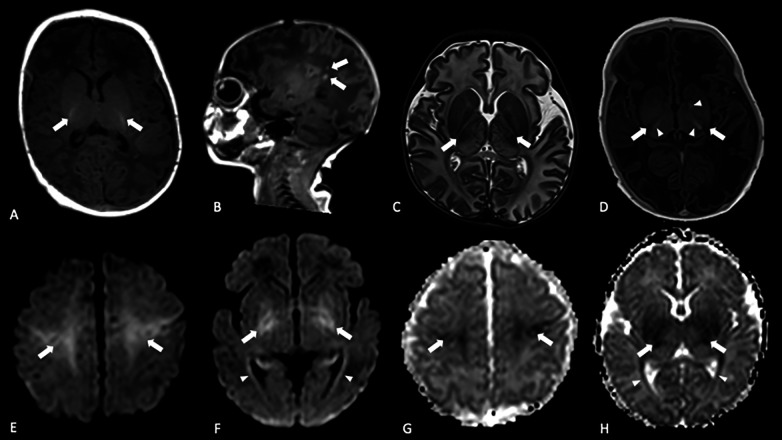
Brain MRI in term HIE neonates treated with TH: (A) axial 3D GrE T1w showing the physiological PLIC hyperintensity (arrows); (B) sagittal 3D GrE T1w demonstrating focal areas of high signal due to PWMLs (arrow);(C) axial TSE T2w showing the absence of the typical PLIC hypointensity (arrows); also, note the abnormal thalamic signal; (D) axial IR T1w showing the loss of the typical PLIC hyperintensity (arrows) and exaggerated hypersignal in the thalami and left globus pallidus (arrowheads); (E,F) axial DWI and (G,H) the corresponding ADC maps showing restricted diffusion in the perirolandic cortex, BGT regions (arrows), and along optic radiation (arrowheads).
